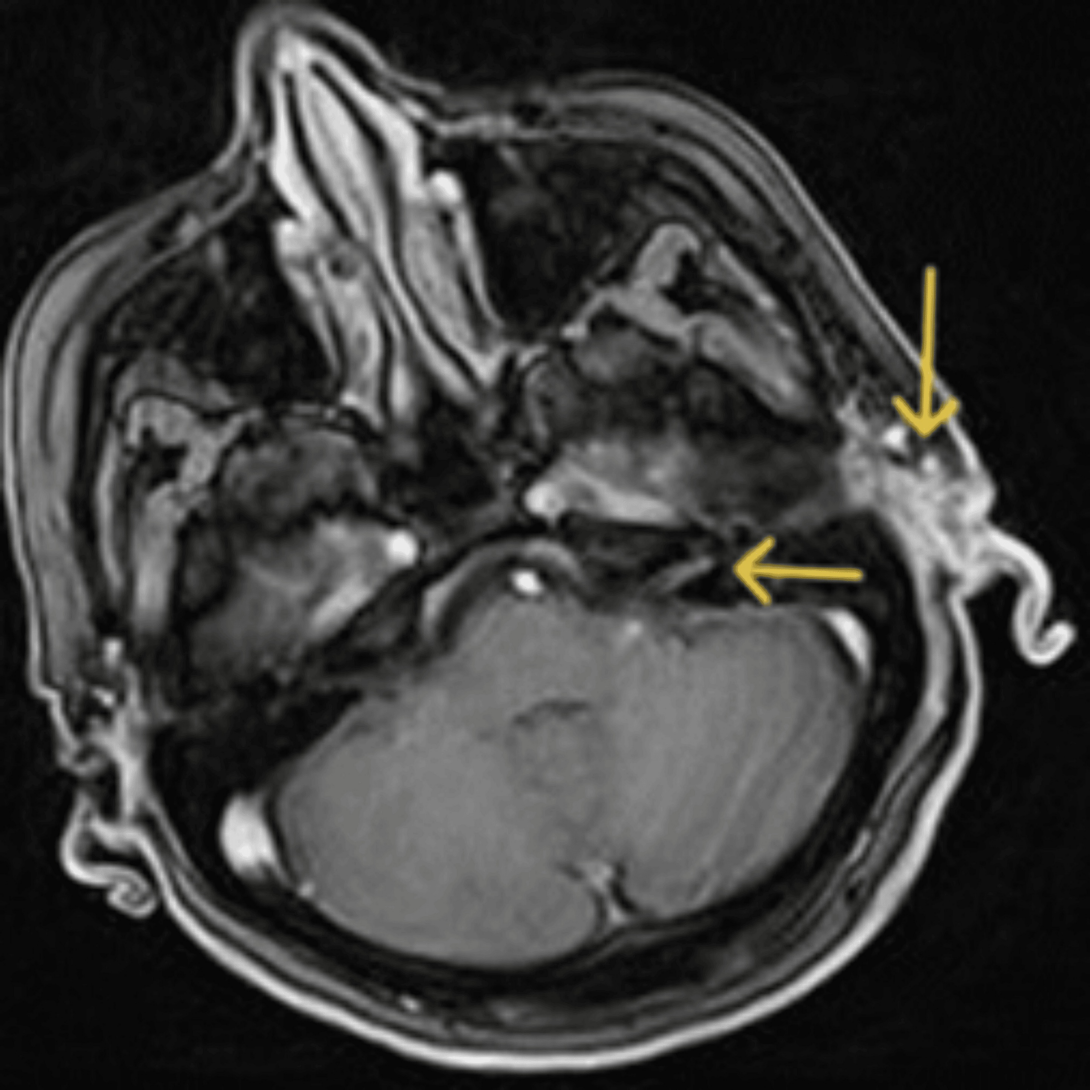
Axial gadolinium-enhanced T1-weighted MRI at the level of internal auditory canals Arrows indicate contrast enhancement within the left internal auditory canal and ipsilateral VII^th^ and VIII^th^ cranial nerves.
